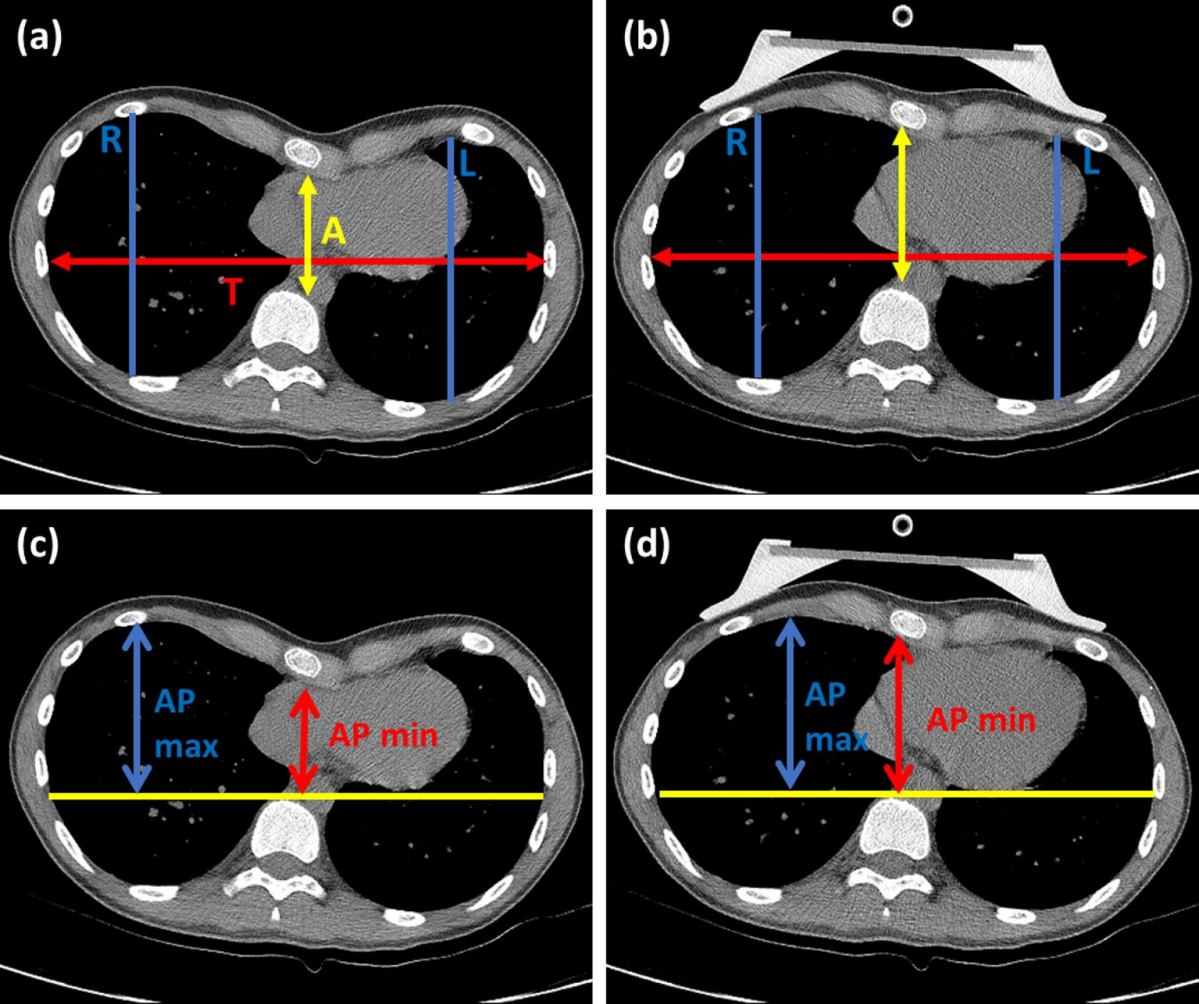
Measurement of asymmetry index (AI) and correction index (CI) using chest computed tomography (CT). (a) Measuring AI before applying vacuum bell (VB) device: The largest sagittal distance of the right (R) and left (L) chest at the same slice on chest CT as that used for HI was used in the formula was AI = R/L, where T is the greater transverse diameter and A is the shortest distance between the vertebrae and sternum. AI > 1.0 implies right asymmetry, while AI < 1.0 indicates left-skewed PE (b) Measurement of AI during application of a VB device: Assessments were performed using the chest CT slice as that used before. (c) Measuring CI before applying a VB device: The minimum distance between the posterior mediastinum and anterior spine (AP min) and maximum distance between the anterior spine and anterior portion of chest wall (AP max) were measured. CI was calculated as follows: CI = ([AP max]-[Ap min])/[AP max]).
